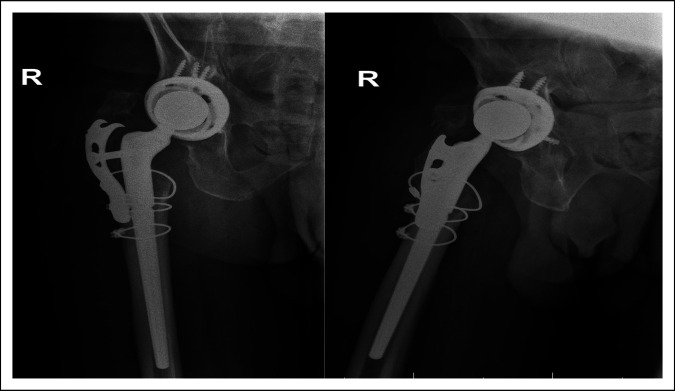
Radiographs from the 3-month follow-up on August 20, 2021.
